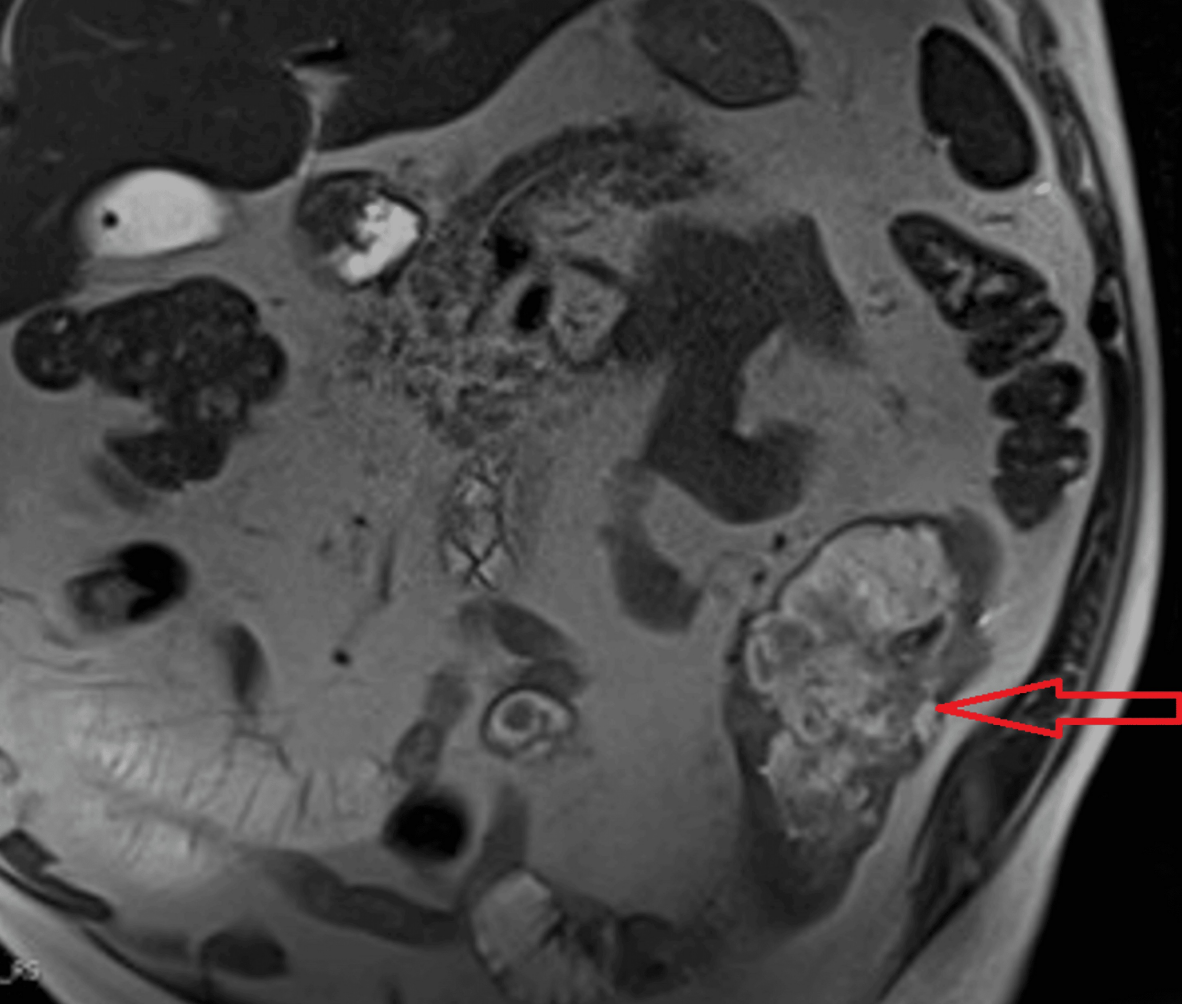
MRI T2 coronal view showing wall thickening and edematous mesentery involving descending colon MRI: magnetic resonance imaging
